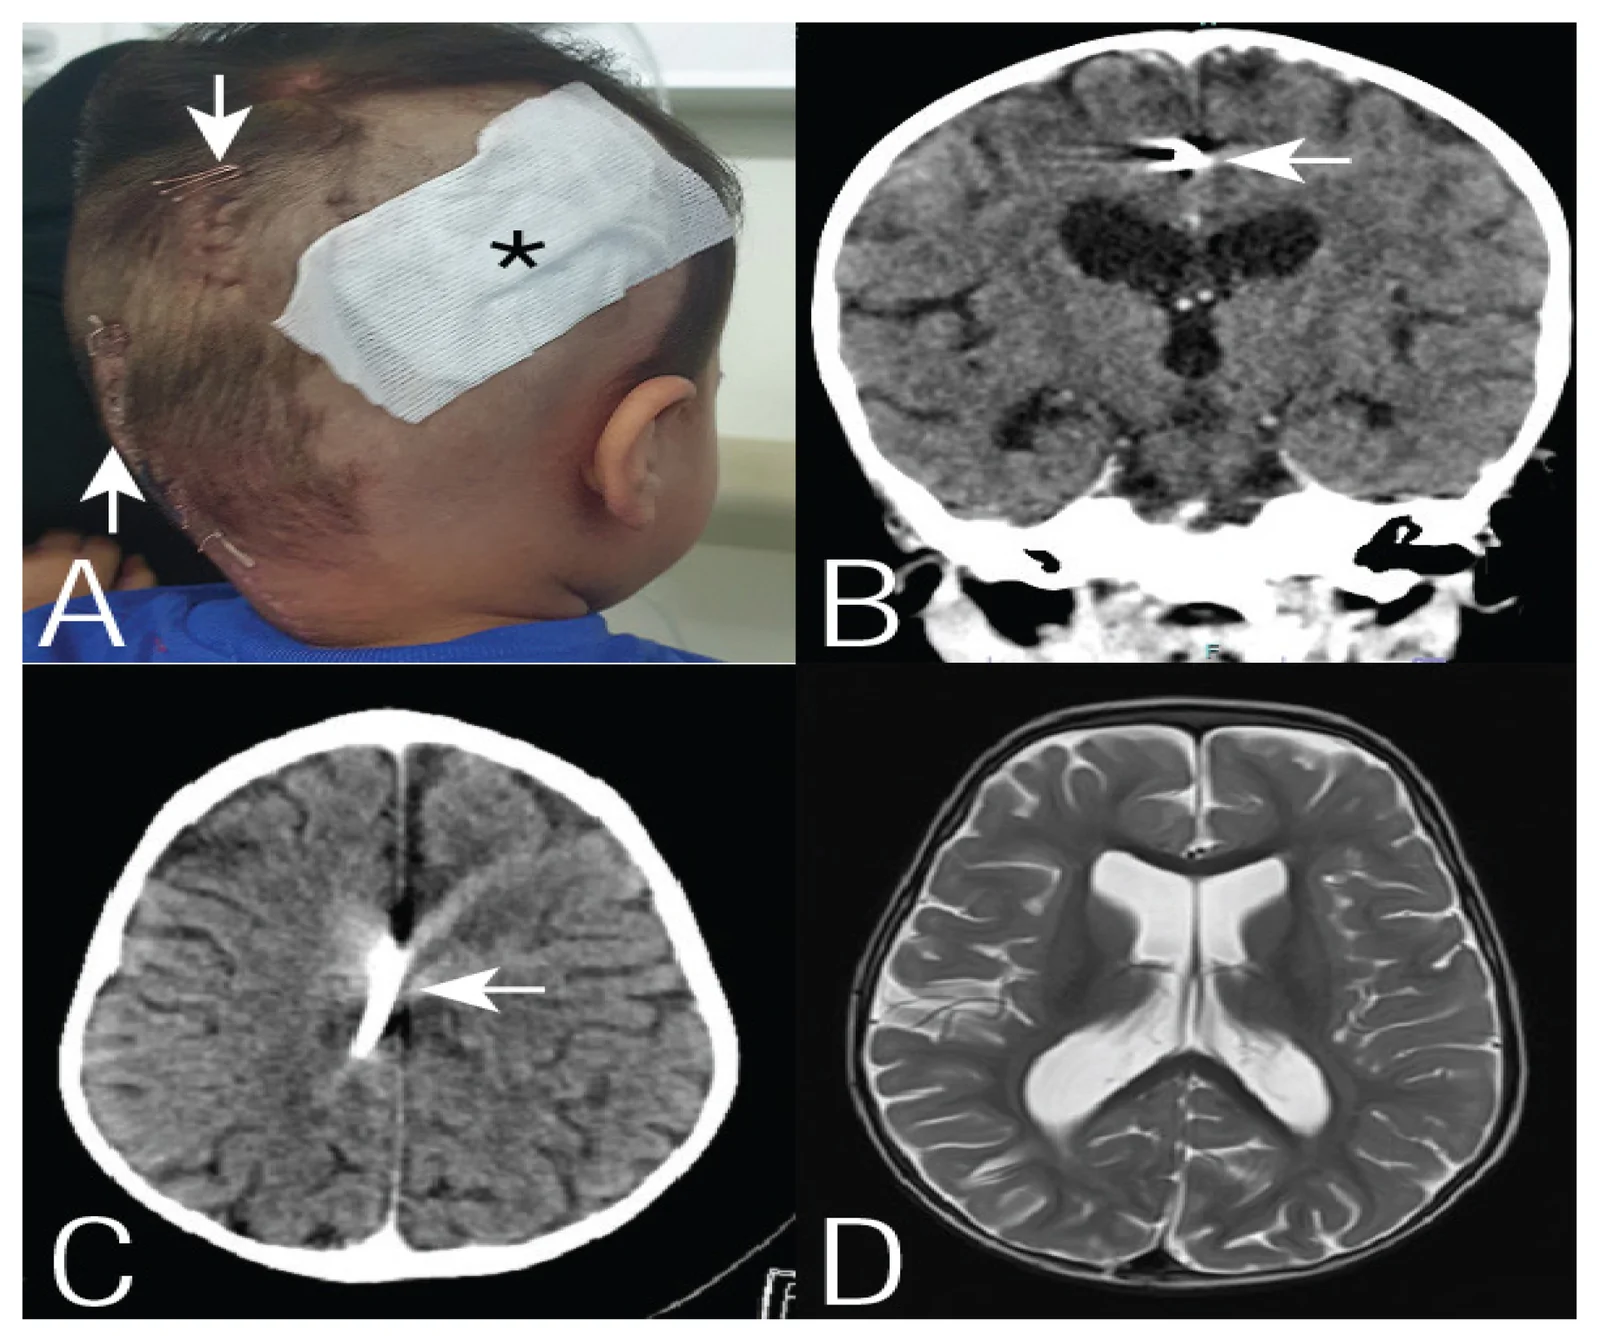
A: A photograph showing complete resolution of pseudomeningocele 24 hours after shunt (arrows) and the dressing over shunt insertion site (asterisk). B & C: Coronal and axial views of a computed tomography scan of the brain after one month showing complete resolution of the subdural hygroma, regression of the hydrocephalus and shunt tube tip in the interhemispheric subdural space (arrows). D: A T2W axial view of a magnetic resonance imaging of the brain after 5 months showing complete resolution of the hydrocephalus and subdural hygroma.
